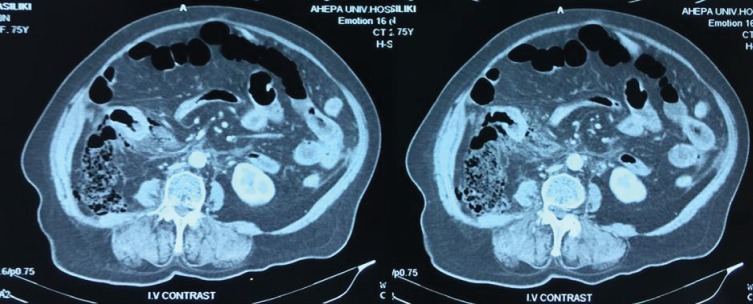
CT scan images showing retrocecal collection of fecal matter, without any presence of free air or fluid collection in the peritoneal cavity.
